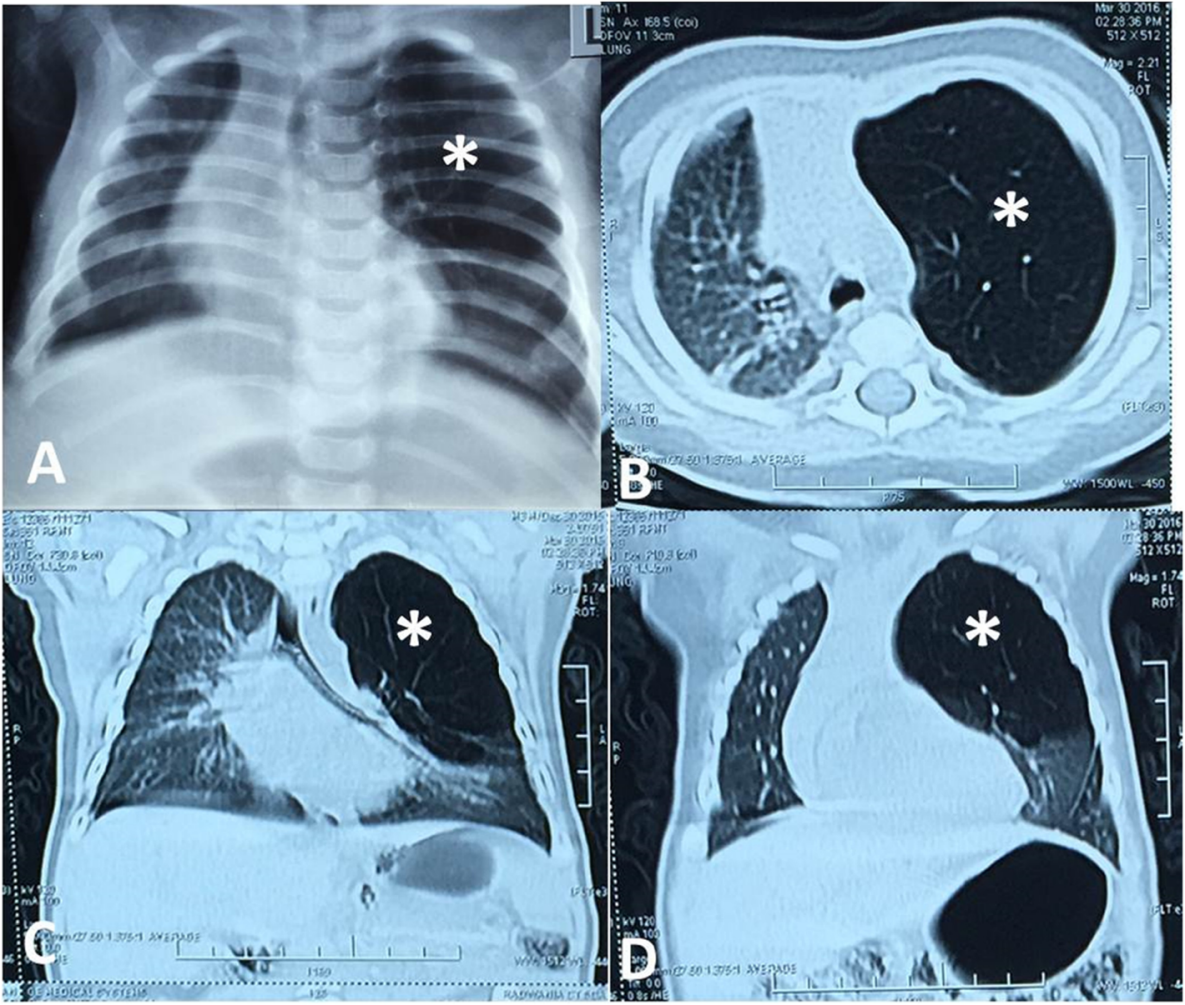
Male infant of 3 months-old has congenital left upper lobe overinflation. a Chest X-Ray, b Axial CT lung window and c&d Coronal CT lung window showing left upper congenital lobar overinflation (*) and mild mediastinal shift
